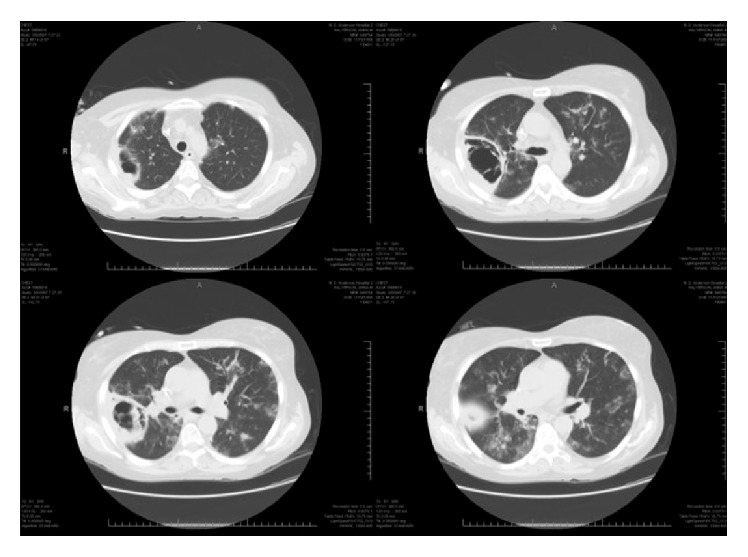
Computed tomography scan sections of the lungs showing progressive enlargement of the right-sided cavitary nodule and the development of new bilateral alveolar opacities.
